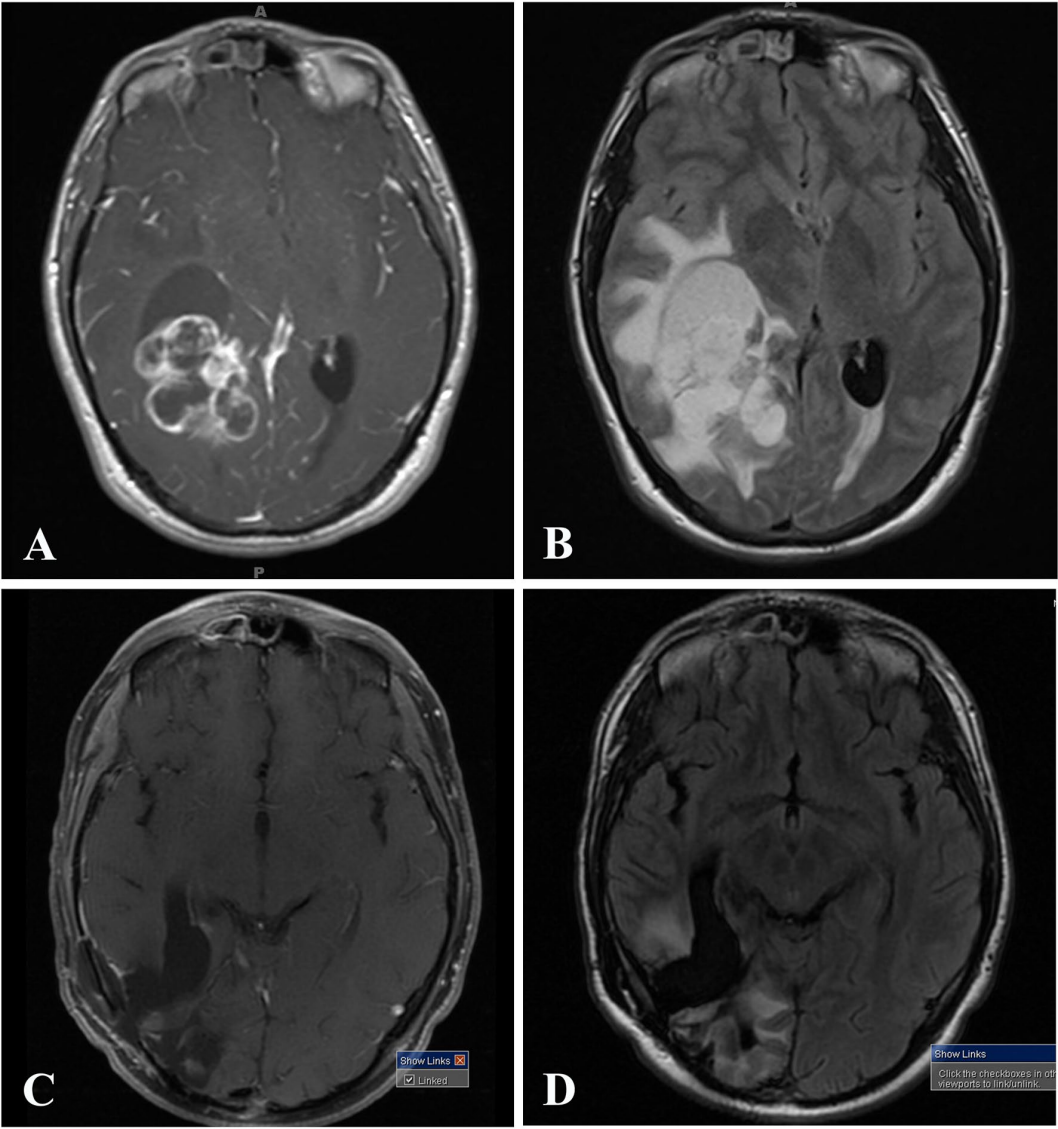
MRI of the brain demonstrating a right-sided, large parieto-temporo-occipital mass, which appeared unusual for a classical GBM, with surrounding vasogenic edema and a right-to-left midline shift. a, b Initial MRI of the brain prior to surgery for tumor resection in January 2015. a T1-weighted post-contrast demonstrating heterogeneous enhancement. b T2-weighted fluid-attenuated inversion recovery (FLAIR) shows significant surrounding vasogenic edema. c, d Status-post resection and two cycles of maintenance TMZ in September 2015. c T1-weighted post-contrast. d Status-post resection, T2-weighted FLAIR sequence
